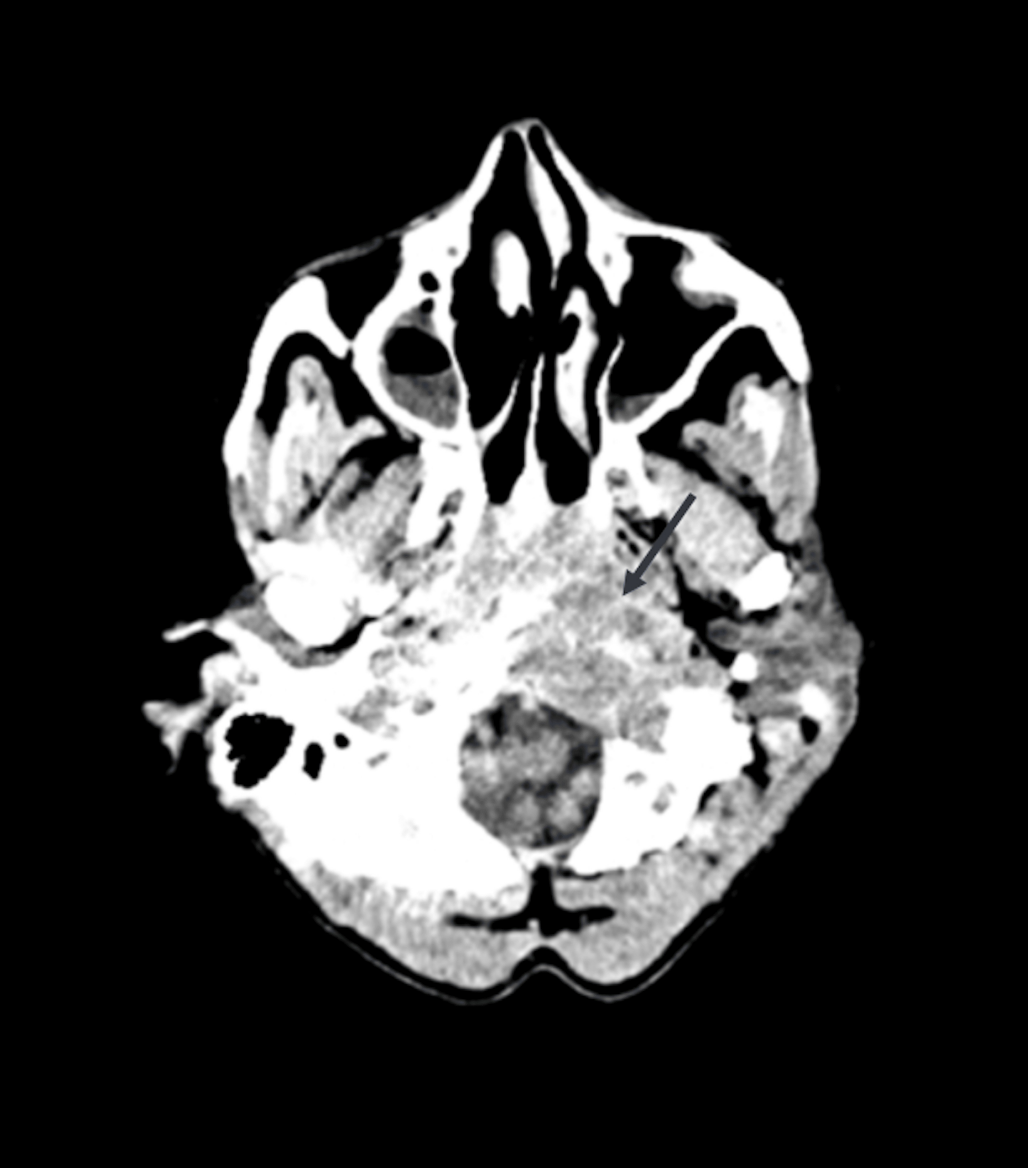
Cranial CT demonstrating extensive osteolysis involving the left occipital condyle and jugular foramen.
